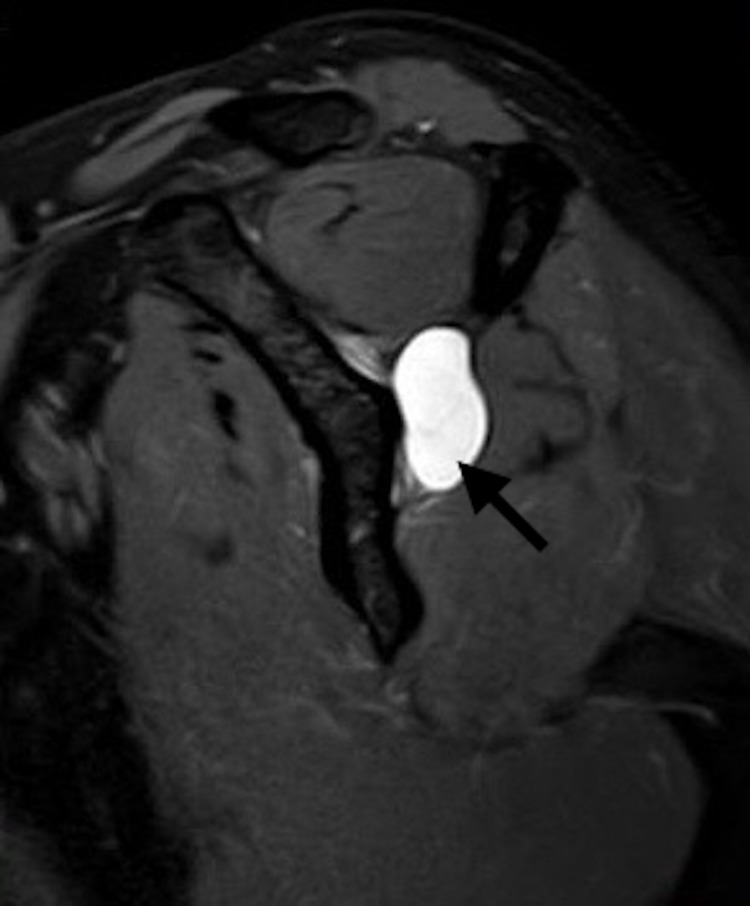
MRI of the right shoulder showing spinoglenoid cyst (arrow)
